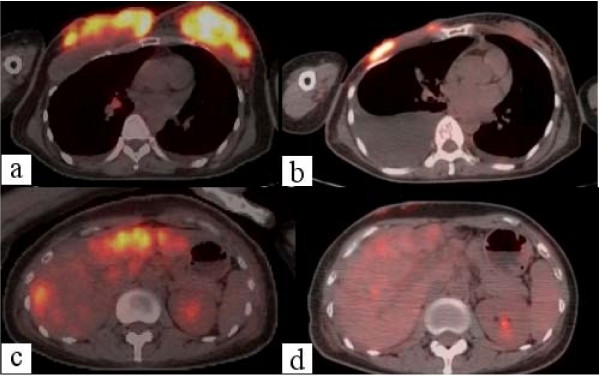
Positron emission tomography/computed tomography fusion images show strong 18F-fluorodeoxyglucose (FDG) uptake in the region of a) the anterior chest wall and b) liver. After chemotherapy, FDG uptake was significantly diminished in the region of c) the anterior chest wall and d) liver.
